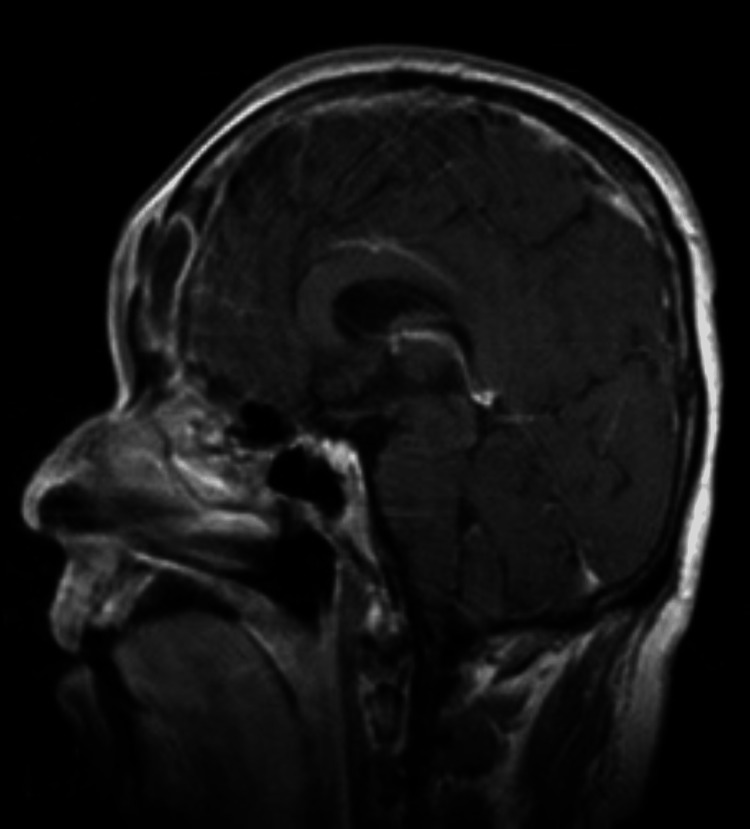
Sagittal T1-weighted post-gadolinium images demonstrating destruction in the interim anterior and posterior walls with intracranial extension as well as extension into the extracranial soft tissues of the frontal region. Fluid collection within the subdural space is also noted (subdural empyema).
